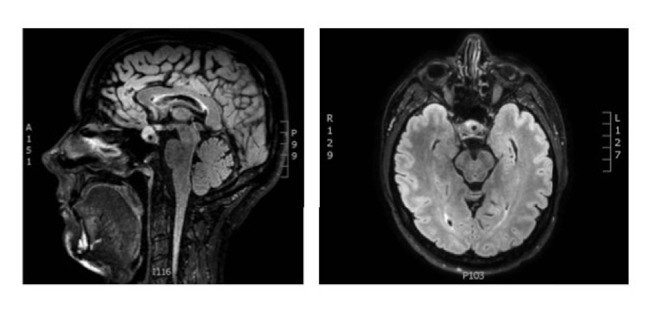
Magnetic resonance imaging of cerebrum. Magnetic resonance imaging (MRI) showed signs of recent bleeding into a cystic adenoma comprising 10x10x8 mm placed in sella turcica with close proximity to the chiasma and displacing the pituitary gland to the right.
